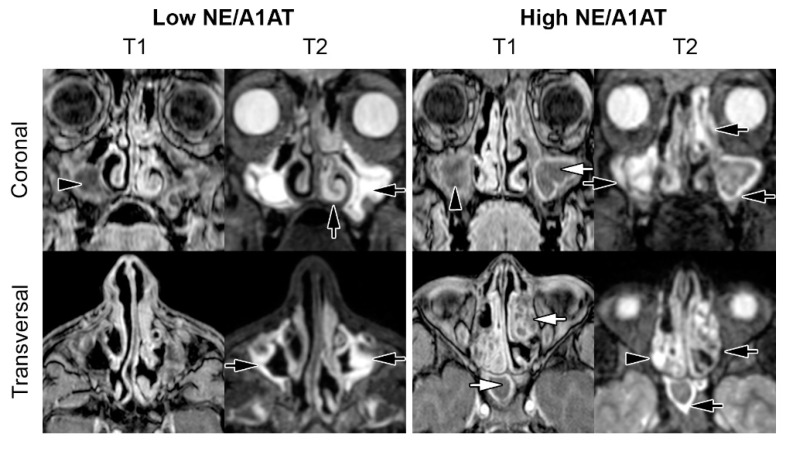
Representative MRI of the paranasal sinuses in patients with CF with low and high NE/A1AT ratios, respectively. A 16-year-old CF patient with a low NE/A1AT ratio demonstrated mucosal swelling (black arrows) of the maxillary sinus and left inferior concha, accompanied by polyps (black arrowheads) in the right maxillary sinus. The CRS-MRI score was 9. The 14-year-old CF patient with high NE/A1AT ratio also showed ubiquitous and more extensive mucosal swelling, polyps, as well as mucopyoceles (white arrows) of the maxillary, ethmoid and sphenoid sinus. The CRS-MRI score was 40. Abbreviations: NE = neutrophil elastase, A1AT = alpha1-antitrypsin.
